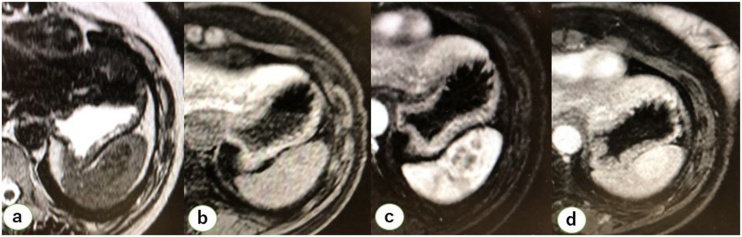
MRI findings. MRI showing a heterogeneous low intensity region within the lesion, indicating fibrosis or hemosiderin, on a T2-weighted image. a) The lesion showed iso-intensity in the spleen, and dynamic study showed gradual enhancement of the lesion [b) 0 s, c) 30 s, d) 180 s] characterized as a “spoke-wheel pattern” on a T1-weighted image.
